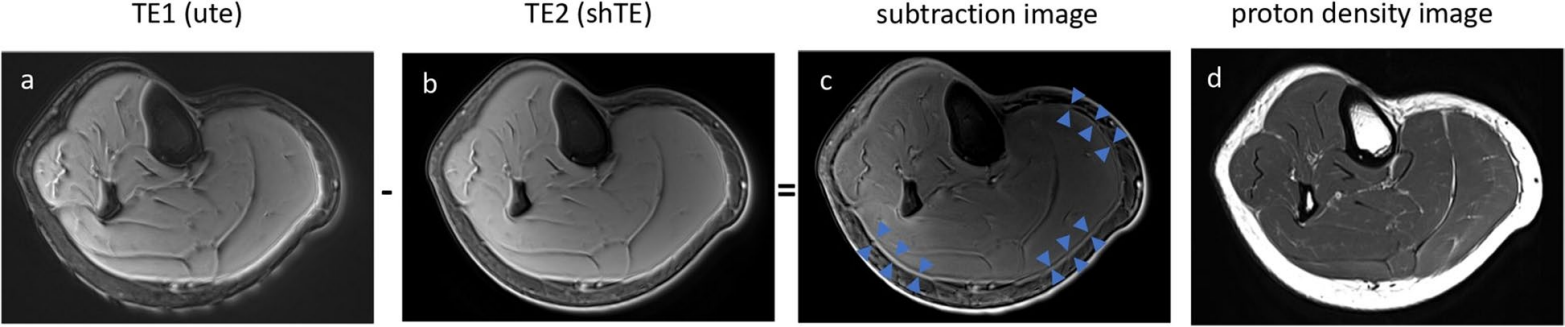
Axial UTE Subtraction and Proton Density Imaging of Lower Leg Post-Injury: Figure (a, b & c) Subtraction of dual echo MR images demonstrates deep fascia tissue with high signal and high contrast (blue arrows in c) whereas figure(d) shows no signal from deep fascia tissue of the leg
